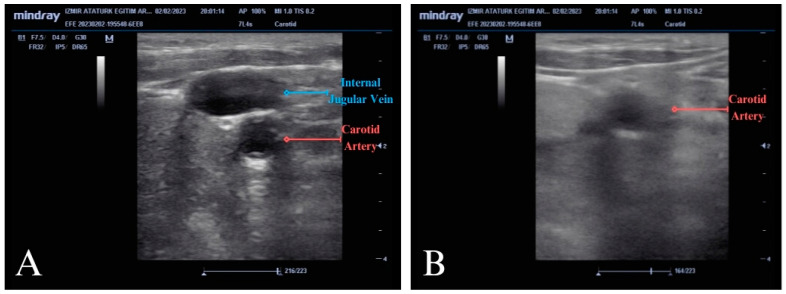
Ultrasound images demonstrating the POCUS-CAC method. (A) Internal jugular vein and carotid artery before applying compression. (B) The image of the carotid artery that does not collapse under POCUS-CAC during chest compressions (early detection of ROSC).
